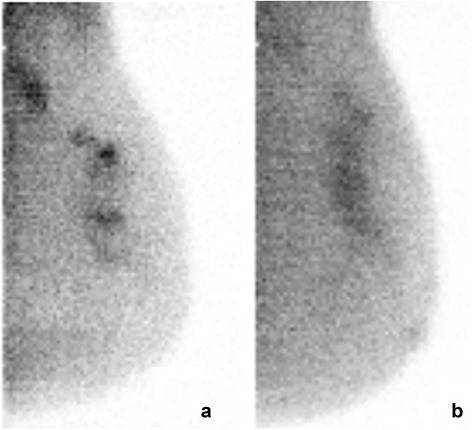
Molecular breast images in the mediolateral oblique projection from a 53-year-old patient with a palpable lesion that was suspicious on diagnostic mammography and scheduled for biopsy (Table 1, tumor #16). At blinded review, MBI performed with 300 MBq ^99m^Tc sestamibi (a) was interpreted as having moderate intensity radiotracer uptake in a segmental distribution with maximum extent of 11.0 cm. An assessment of 4 was assigned. MBI performed 1 day later with 300 MBq ^99m^Tc maraciclatide (b) was also interpreted as assessment 4 with lesion extent measuring 9.2 cm. Pathology revealed grade III invasive ductal carcinoma. Patient underwent subsequent neoadjuvant chemotherapy. Tumor extent measured on contrast-enhanced MRI prior to neoadjuvant chemotherapy was estimated at 9.2 cm
